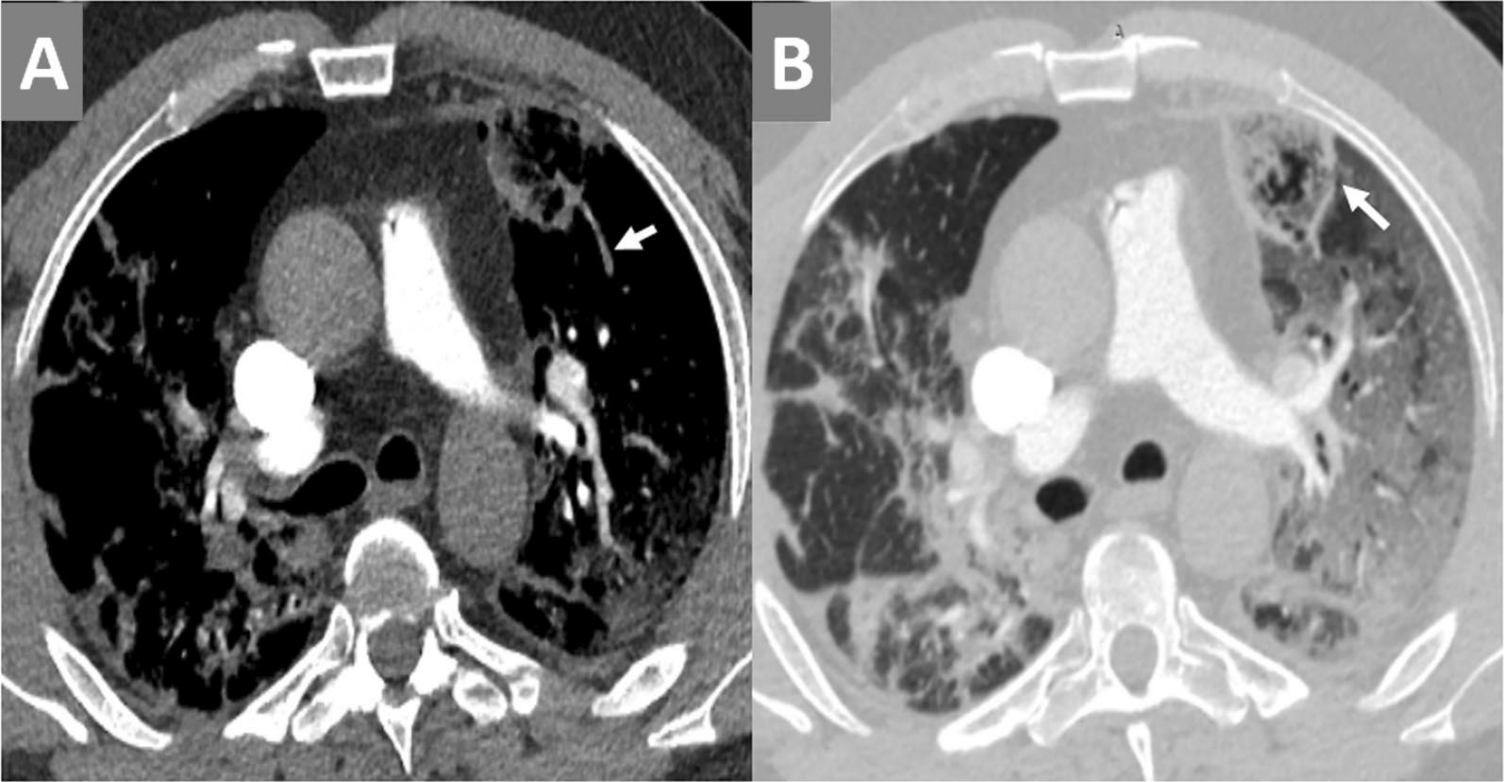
CT-pulmonary angiography (CTPA) examination for a 69-years-old COVID-19 male patient complained of severe chest pain and sudden deterioration of oxygen saturation in the second week of infection. [A] Axial CT image with MIP reconstruction showed left upper lobar peripheral acute pulmonary embolism (white arrow) and nearby sub-pleural wedge-shaped pulmonary infarction. [B] Axial CT image (lung window) showed the class (atoll-sign) of the pulmonary infarction (white arrow) and typical sub-pleural ground-glass bilateral patches of COVID-19 infection
